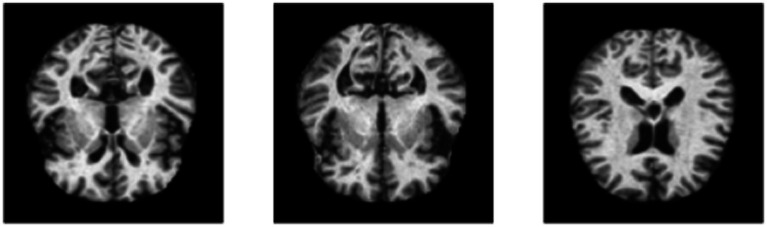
MRI image labeling before diagnosing the disease.
